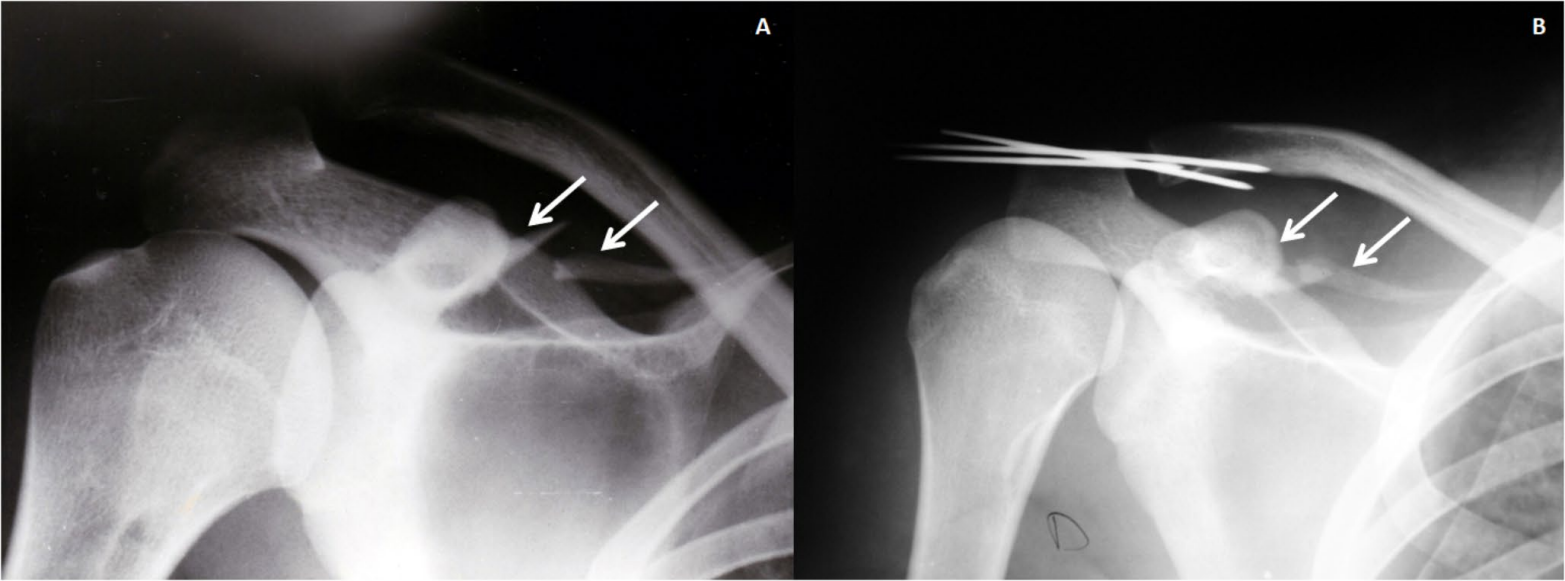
Case 6 Table 1. A 19 years-old girl: 2A: Antero-posterior radiograph showing an AC separation with and CP base and superior border of the scapula fracture; 2B: Treatment was made with an AC fixation with Kirschnner wires without surgical fixation of the CP
